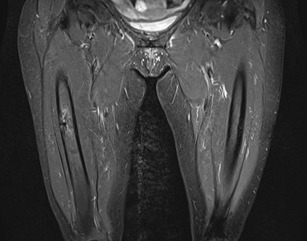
MRI Scan Showing Incomplete Atypical Femoral Fracture with Edema and a Lateral Fracture Line.
